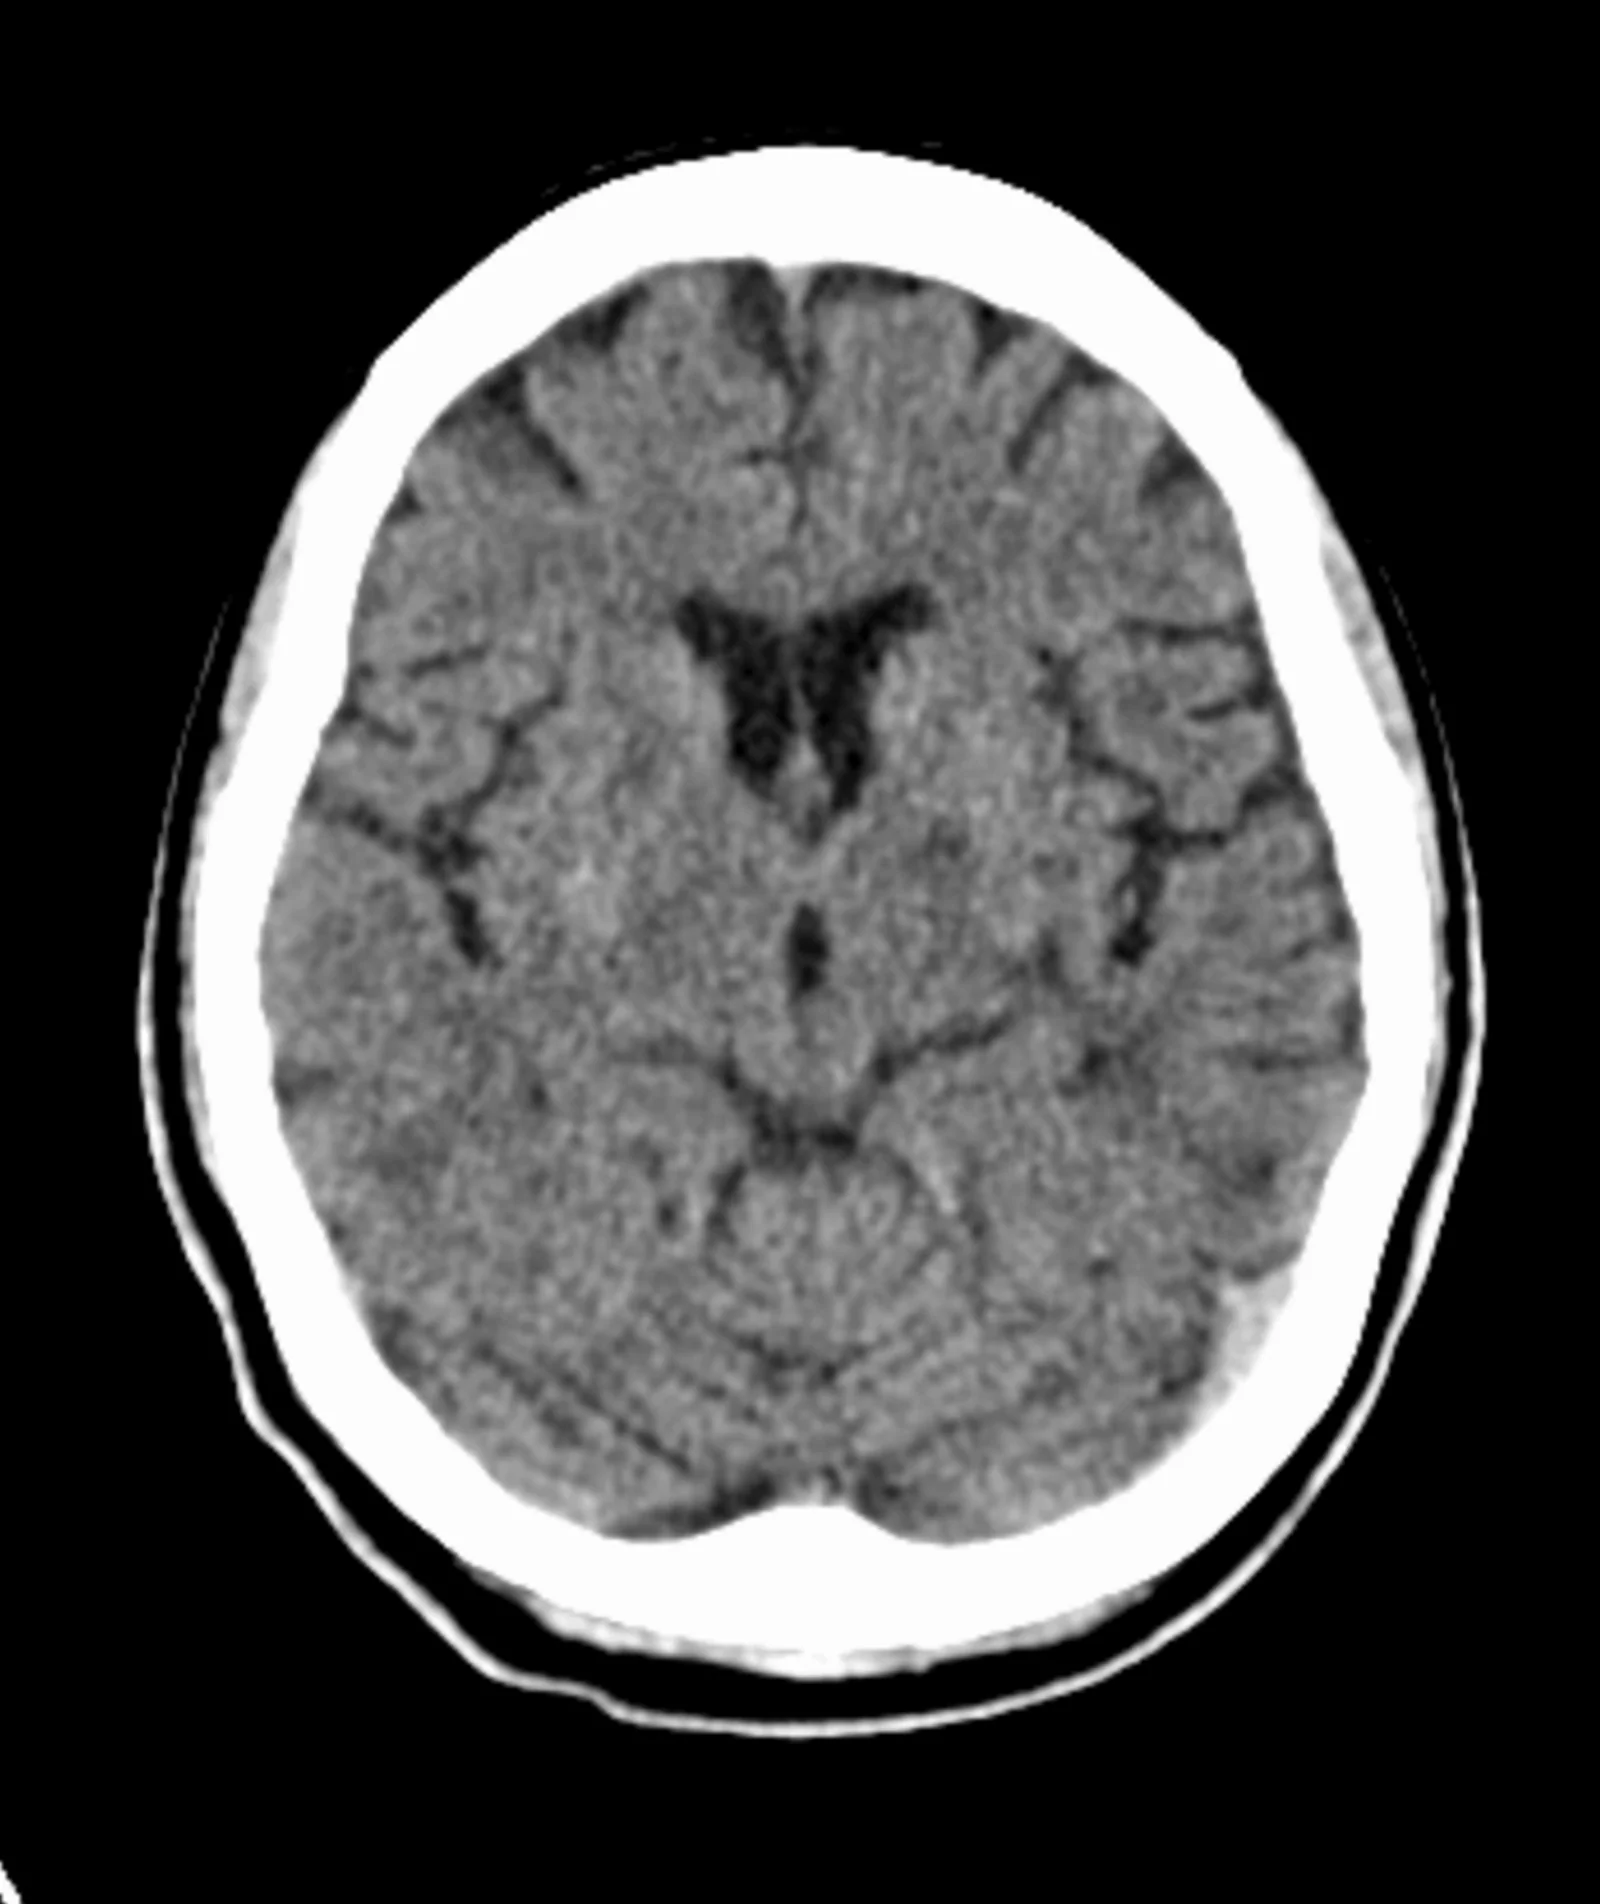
Head CT. Head CT scan with no acute intracranial abnormality. Mild cerebral atrophy can be noted.
